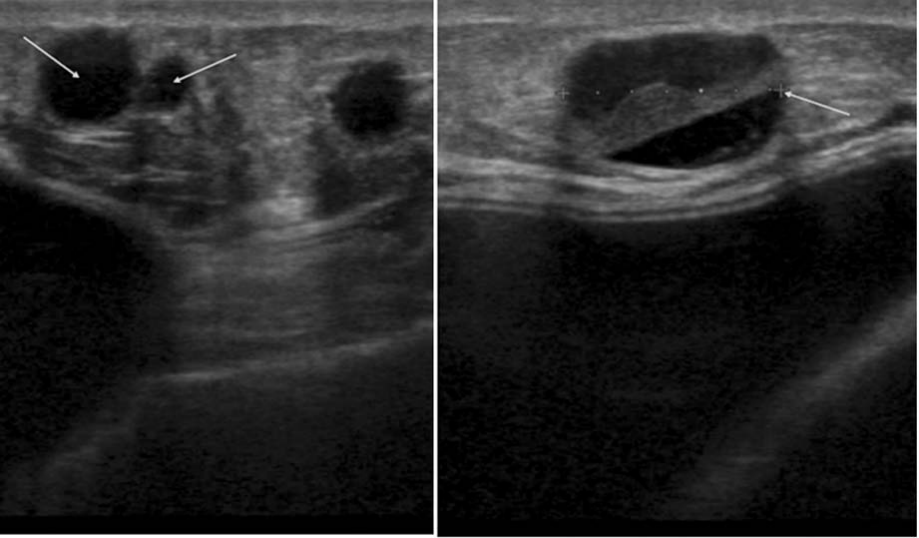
Post mastectomy LD and implant based reconstruction of right breast, had fat grafting (270 ml) in 2009. Clinical manifestation of multiple palpable nodules in second postoperative year. Ultrasound right reconstructed breast showed multiple well defined lesions in the subcutaneous fat consistent with fat necrosis and oil cysts, arrows shows cysts of varied sizes with largest 15 mm reported as U2.
